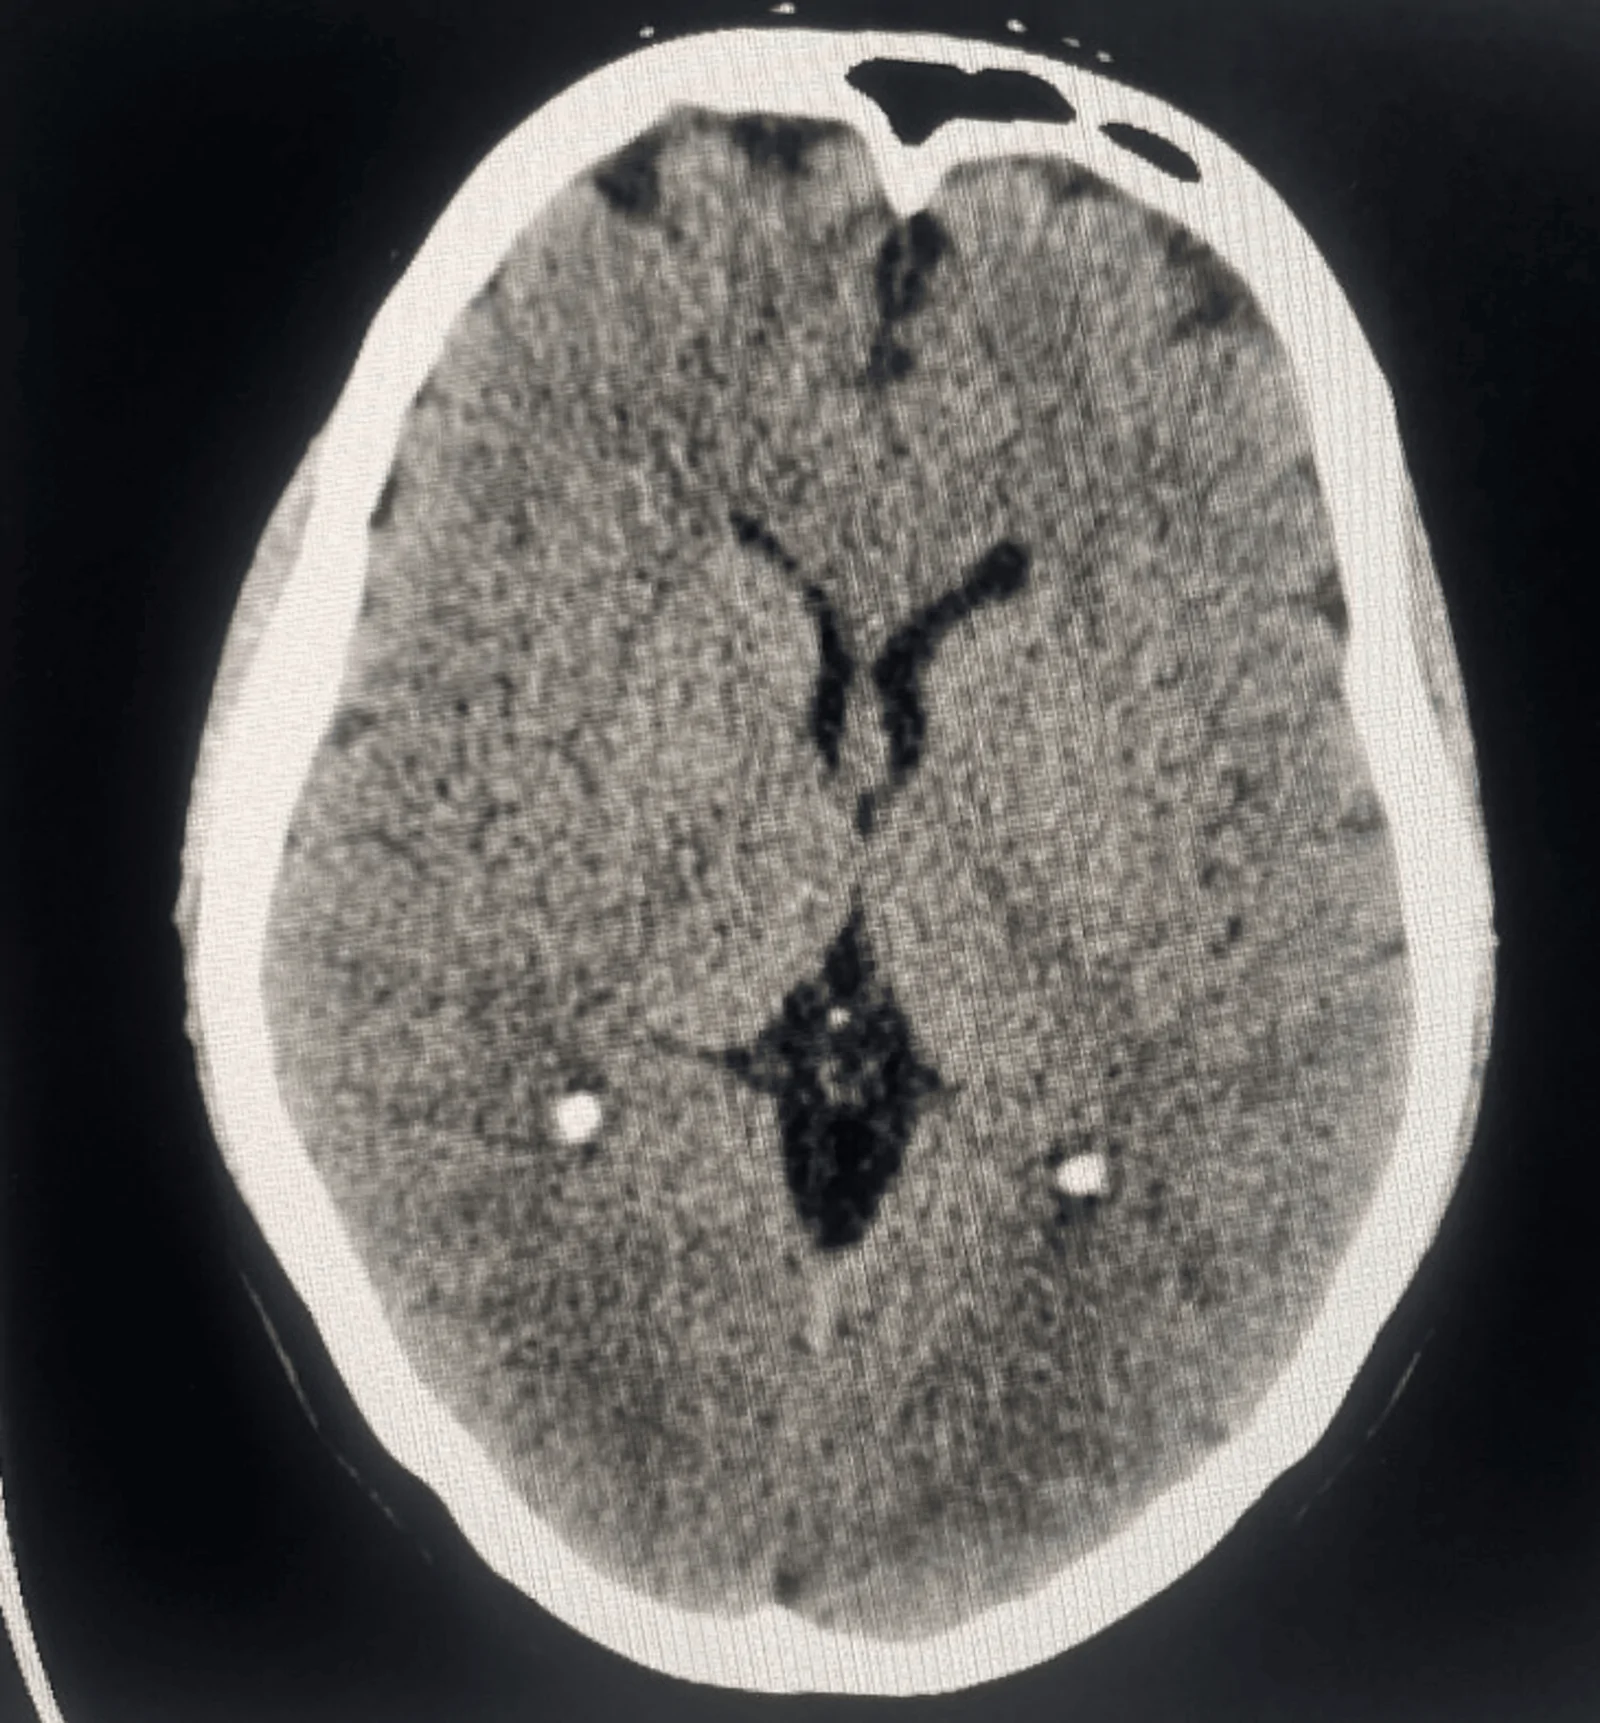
Computed tomography scan of the brain showing no acute intracranial pathology Axial non-contrast computed tomography image demonstrating no evidence of acute intracranial pathology, including intracranial hemorrhage, infarction, mass effect, or hydrocephalus
